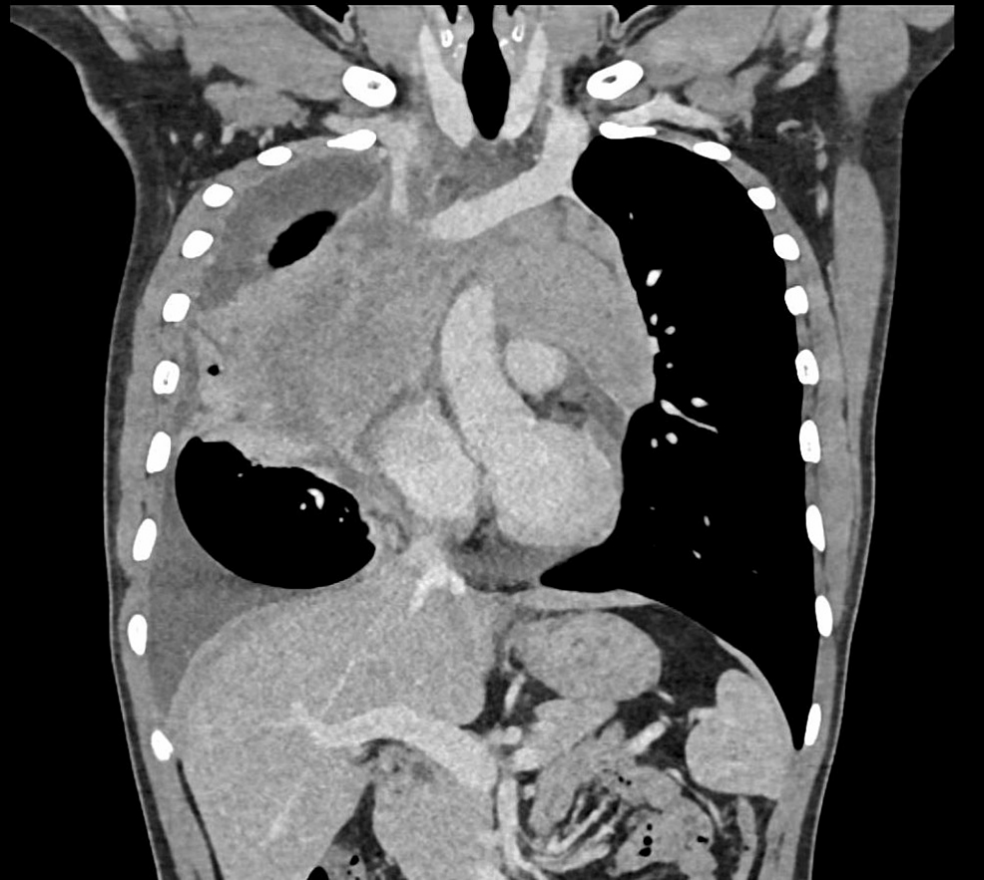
Contrast-enhanced computed tomography scan of the thorax-coronal section shows a lesion extending anterio-superiorly from the level of the sternoclavicular joint to the level of the T6 vertebral body inferiorly. The lesion extends into the aortopulmonary window and encases the arch of the aorta, ascending aorta, main pulmonary trunk, superior vena cava, and left brachiocephalic vein for an arc of 270 degrees. It encases the right and left main pulmonary arteries for an arc of 180 degrees and is also seen abutting the right and left upper lobe segmental bronchi and arteries, and bilateral superior and inferior pulmonary veins.
